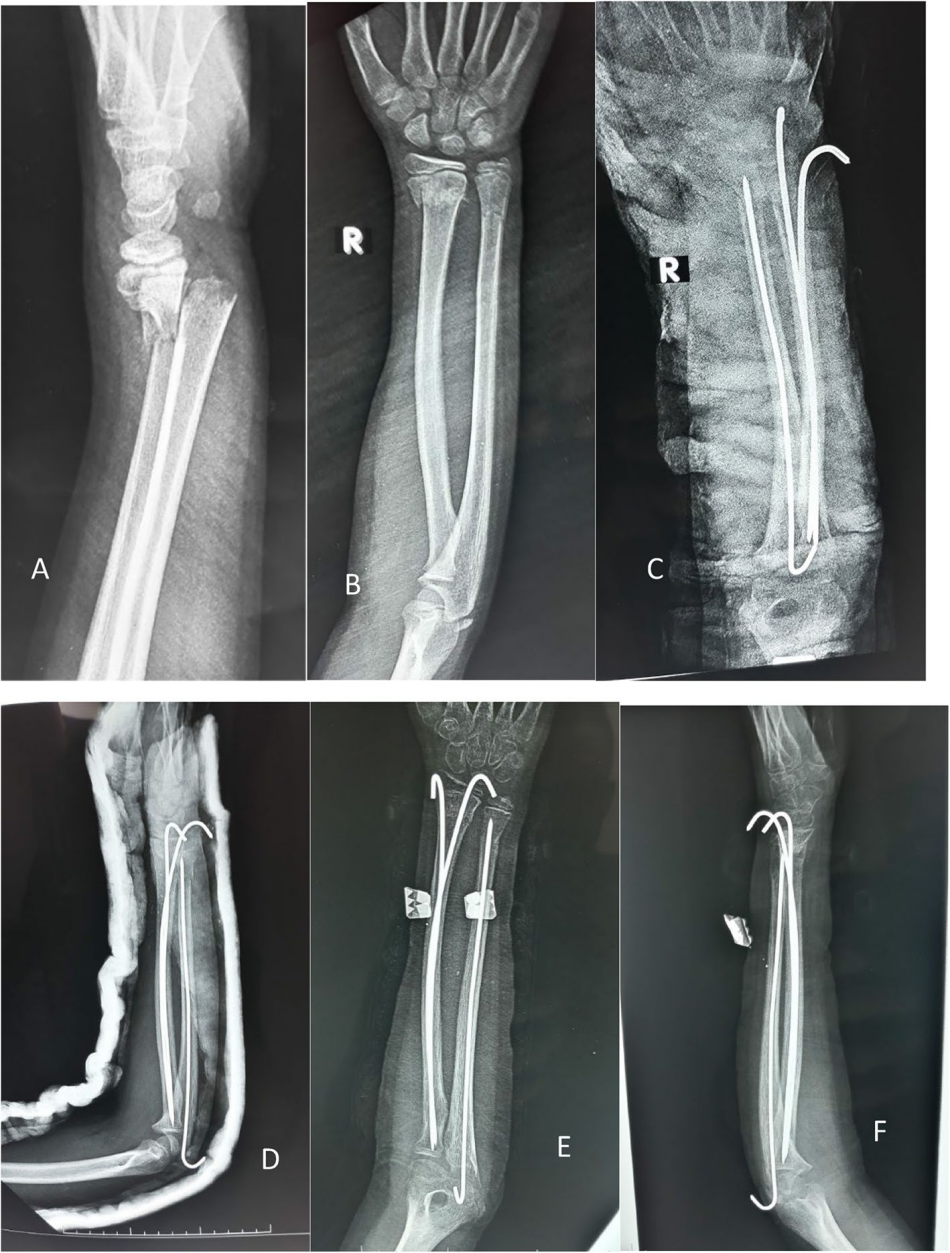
A and B x-rays show Anteroposterior and lateral x-rays show a fracture distal forearm in a female 9-year-old patient. C and D postoperative X-rays. E and F x-rays after 6 weeks show stable fixation with a solid union
